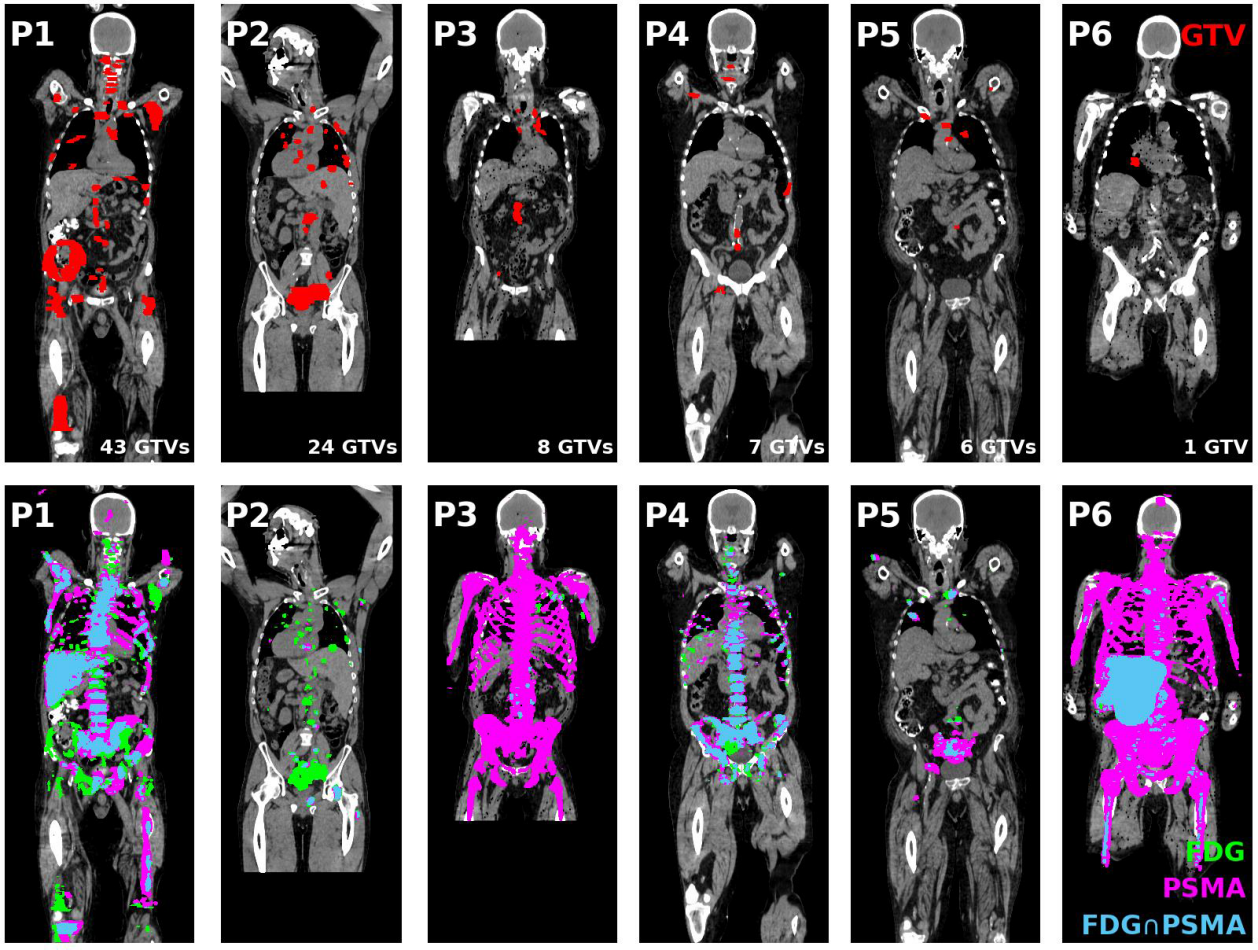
(Top tow) GTV contours (red) of all coronal slices projected on the middle coronal slice for all patients included in this study. (Bottom row) FDG (green) and PSMA (magenta) PET distribution and their intersection (blue) projected on the middle coronal slice for each patient. GTV, Gross tumour volume; FDG, ^18^F-fluorodeoxyglucose; PSMA, Prostate specific membrane antigen; PET, Positron emission tomography.
